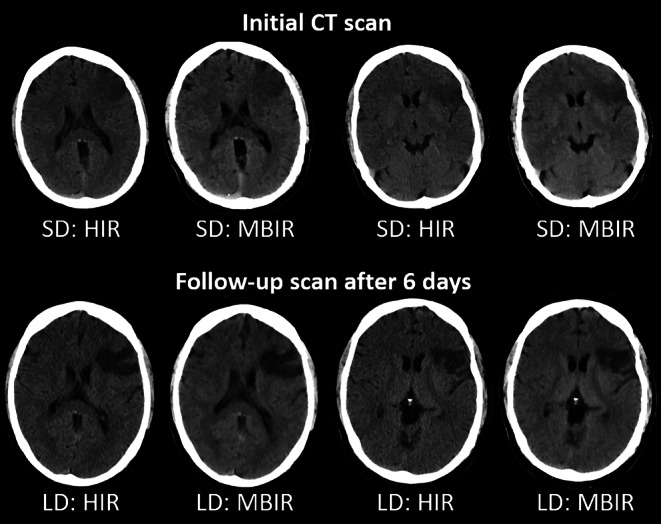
Initial SD NCCT (*upper row*) and follow-up LD NCCT (*lower row*) of a 41-year-old man with left-sided MCA infarction. Axial images were reconstructed using HIR and MBIR. The demarcated area in the left hemisphere shows better conspicuousness due to higher CNR with MBIR compared to HIR. *CNR* contrast-to-noise ratio, *HIR* hybrid iterative reconstruction, *LD* low-dose, *MBIR* model-based iterative reconstruction, *MCA* middle cerebral artery, *NCCT* non-contrast computed tomography, *SD* standard-dose
